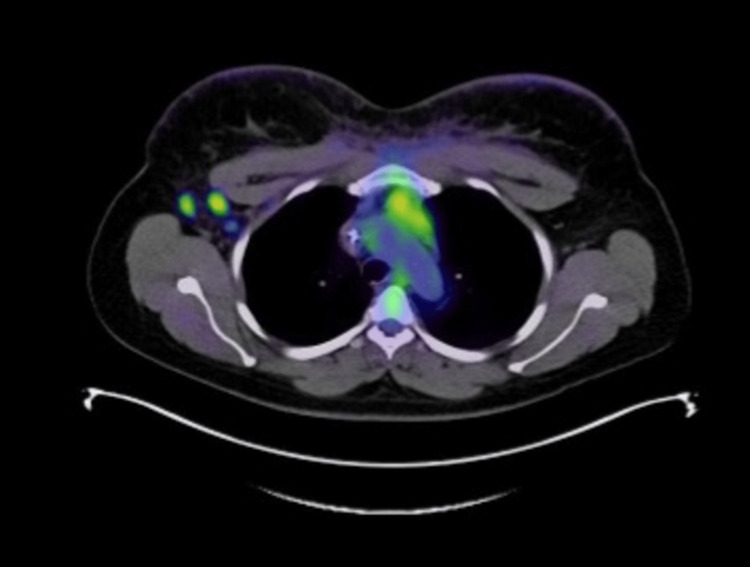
Axial PET/CT image demonstrating hypermetabolic uptake in the right axilla. PET: positron emission tomography, CT: computed tomography
